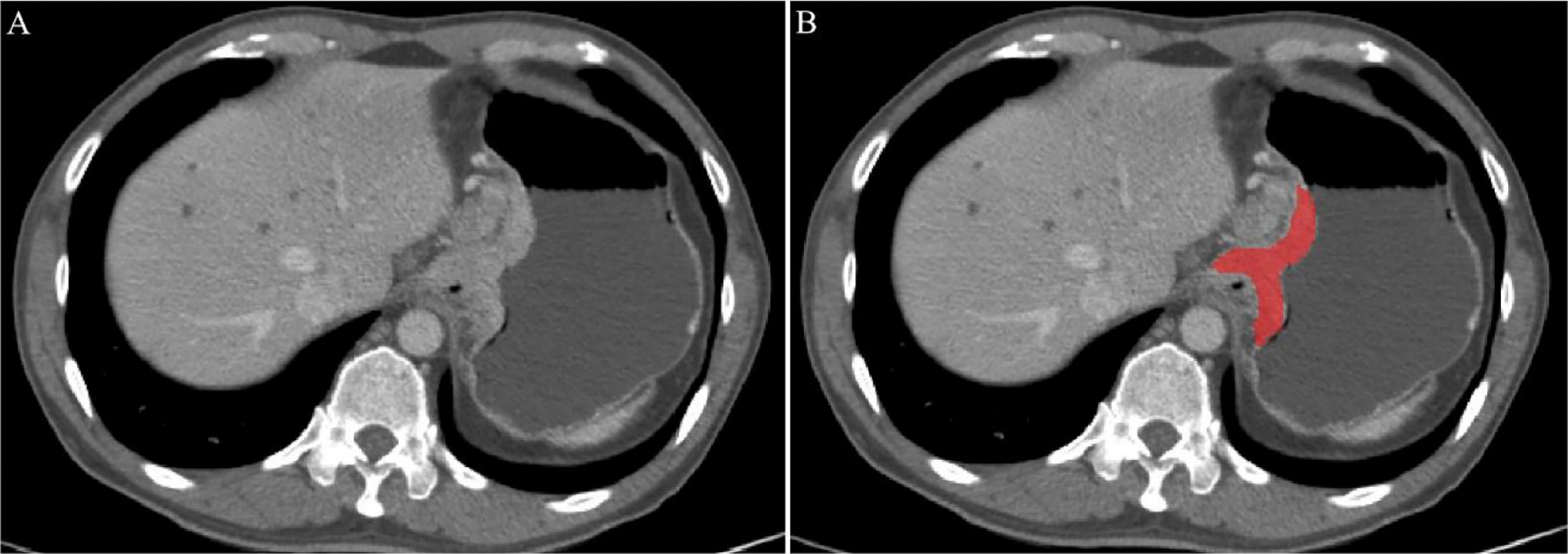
An example of manual segmentation in Adenocarcinoma of the esophagogastric junction (AEG). (A) Localized thick wall of esophagogastric junction with enhancement is observed on the portal venous phase computed tomography (CT) image; (B) Segmentation of the same axial slice.
